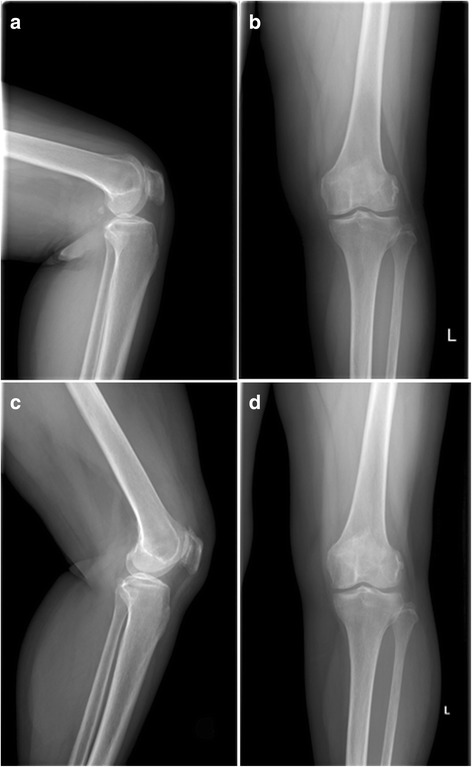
a-b Preoperative and c-d postoperative X-ray films of the patients
